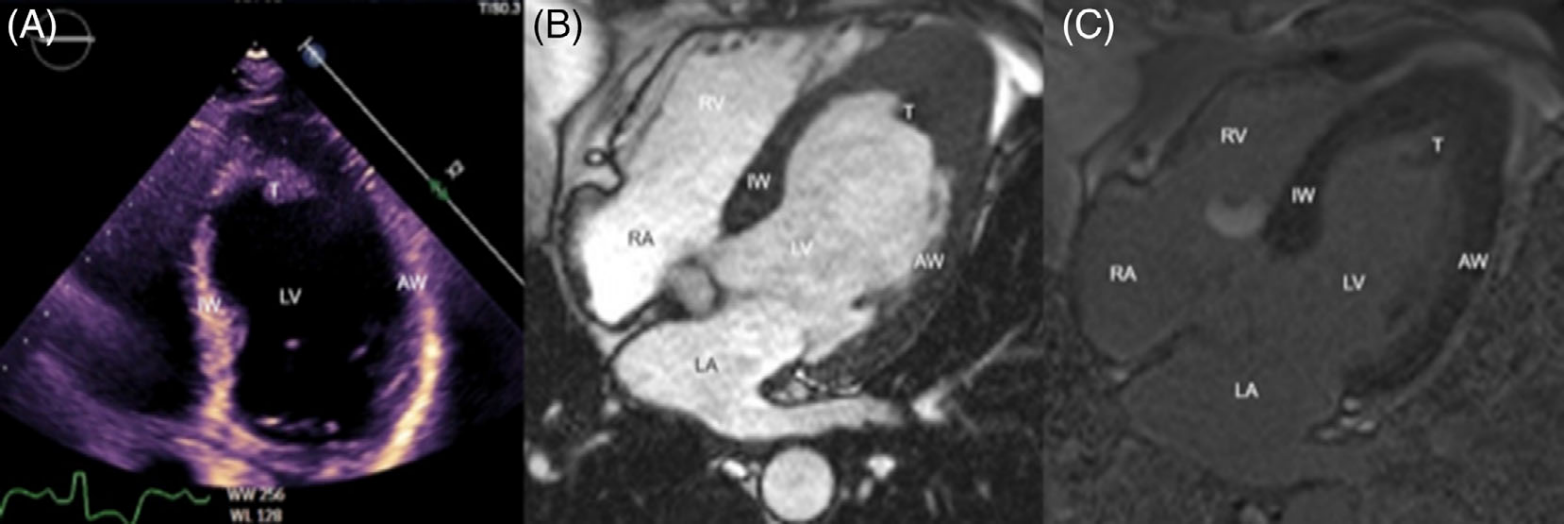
Apical 4 chamber view on transthoracic echocardiogram (A) and cardiac magnetic resonance (cMRI) imaging pre gadolinium cine image (B) and post gadolinium (C). IW, inferoseptal wall; AW, anterolateral wall; T, thrombus; LV, left ventricle; LA, left atrium
